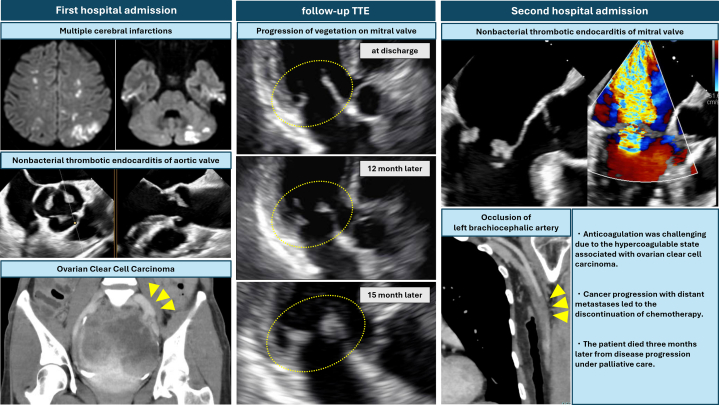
Recurrent NBTE With Systemic Embolic Events TTE = transthoracic echocardiography.
